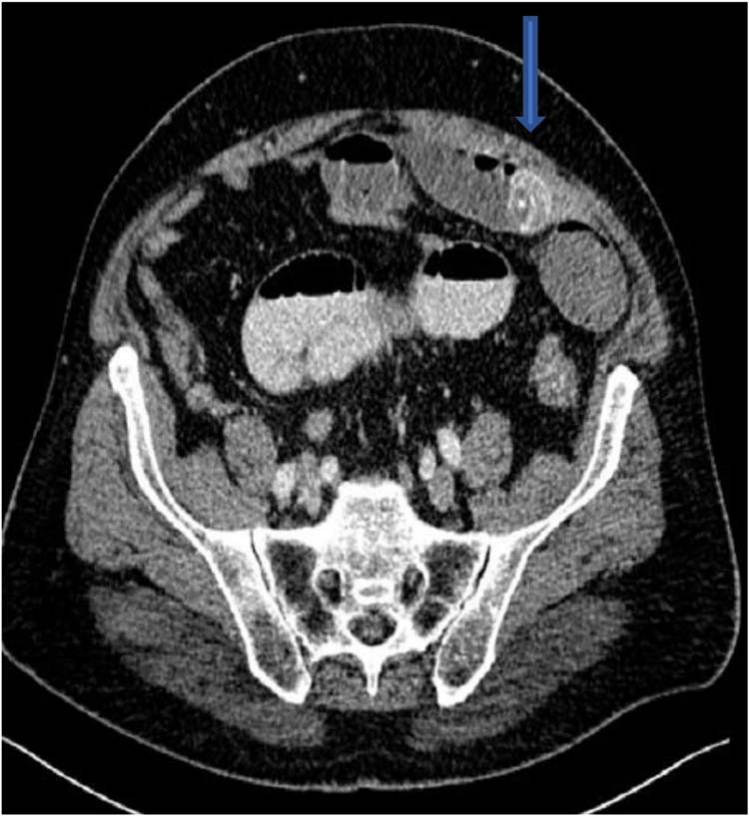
CT showing stone in distal jejunum.
